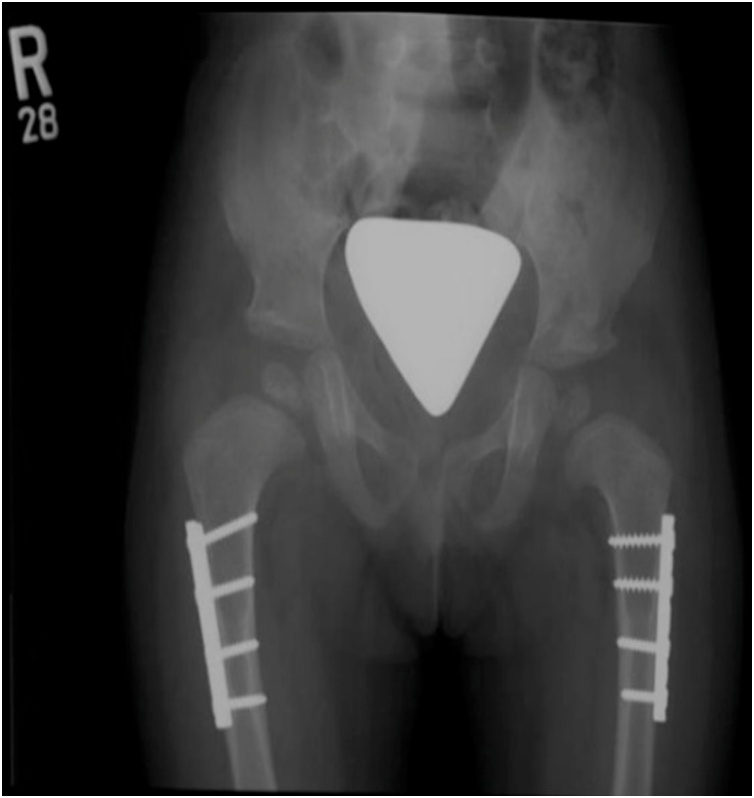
Pelvis AP x-ray at age of 3 years after bilateral hips open reduction, adductor tenotomy, pelvis osteotomy, femoral shortening and hip Spica, Right hip 12 months post- operatively, left hip 6 months post-operatively.
